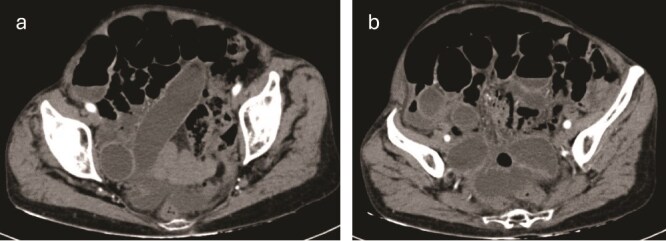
(a, b) Contrast-enhanced CT of the abdomen revealed loops of small intestine with dilation up to 31 mm and the presence of a whirl sign at the level of the distal ileum associated with a transition zone.
